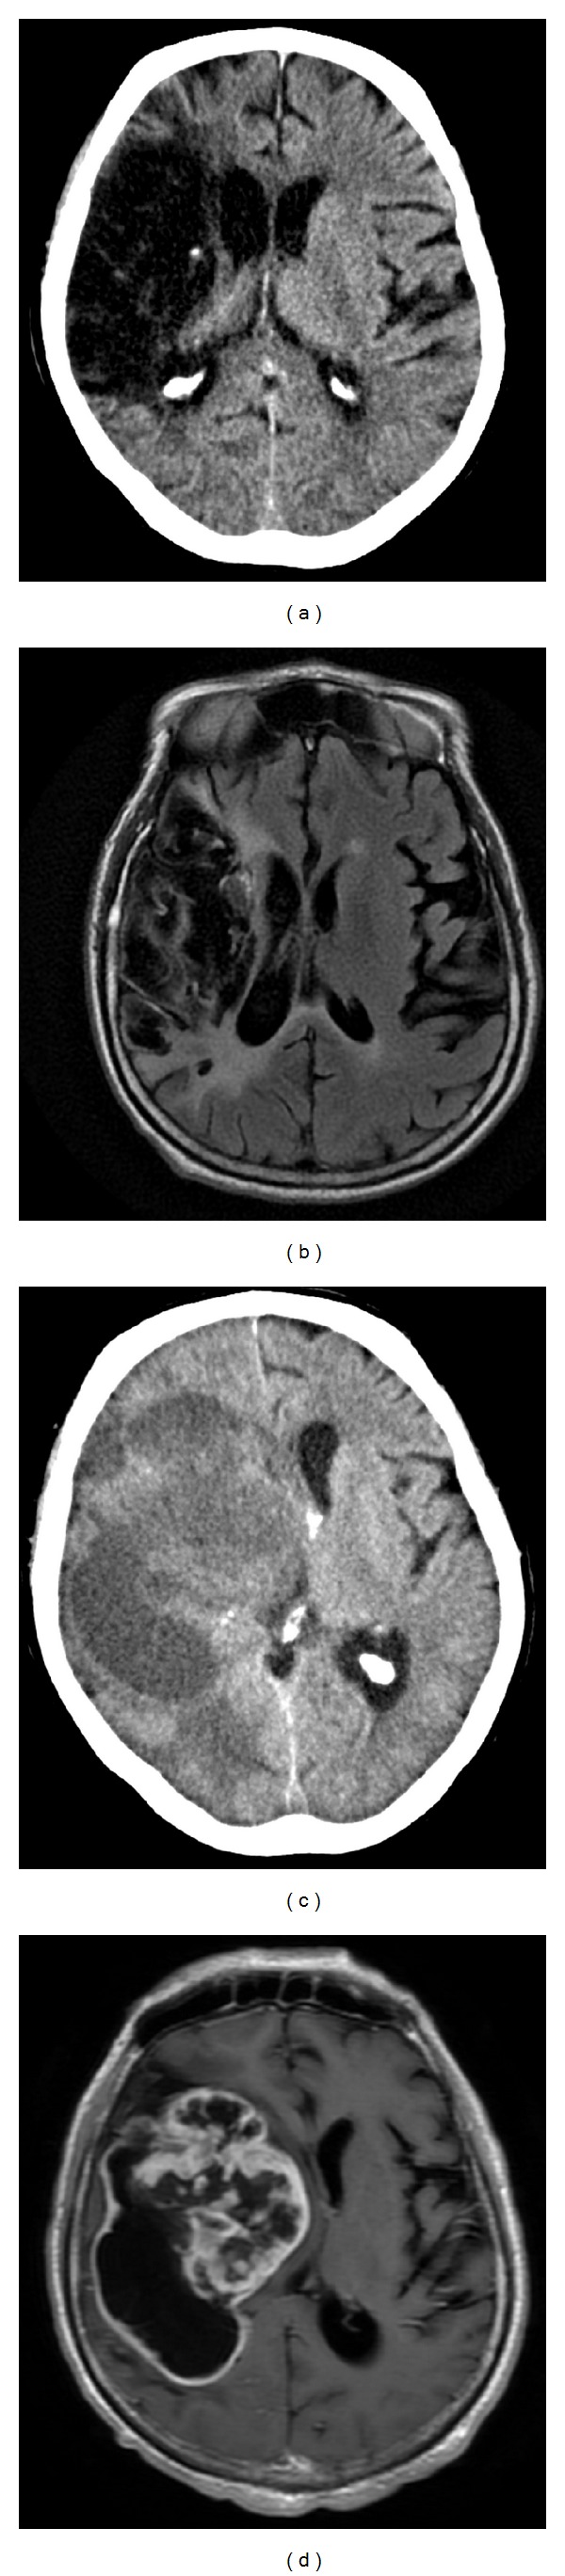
Evolution of gliotic scar after ischemic stroke. (a) CT without contrast 17 months after ischemic stroke, showing a gliotic scar. (b) MRI FLAIR sequence 17 months after ischemic stroke, showing gliotic scar within the previously infarcted region. (c) CT without contrast two years after ischemic stroke, showing a dense, cystic area in the right frontal, temporal, and parietal lobes, corresponding to the region of the prior infarct. (d) MRI T1 sequence after gadolinium contrast two years after ischemic stroke, showing contrast-enhancing, necrotic mass in the previously infarcted region.
